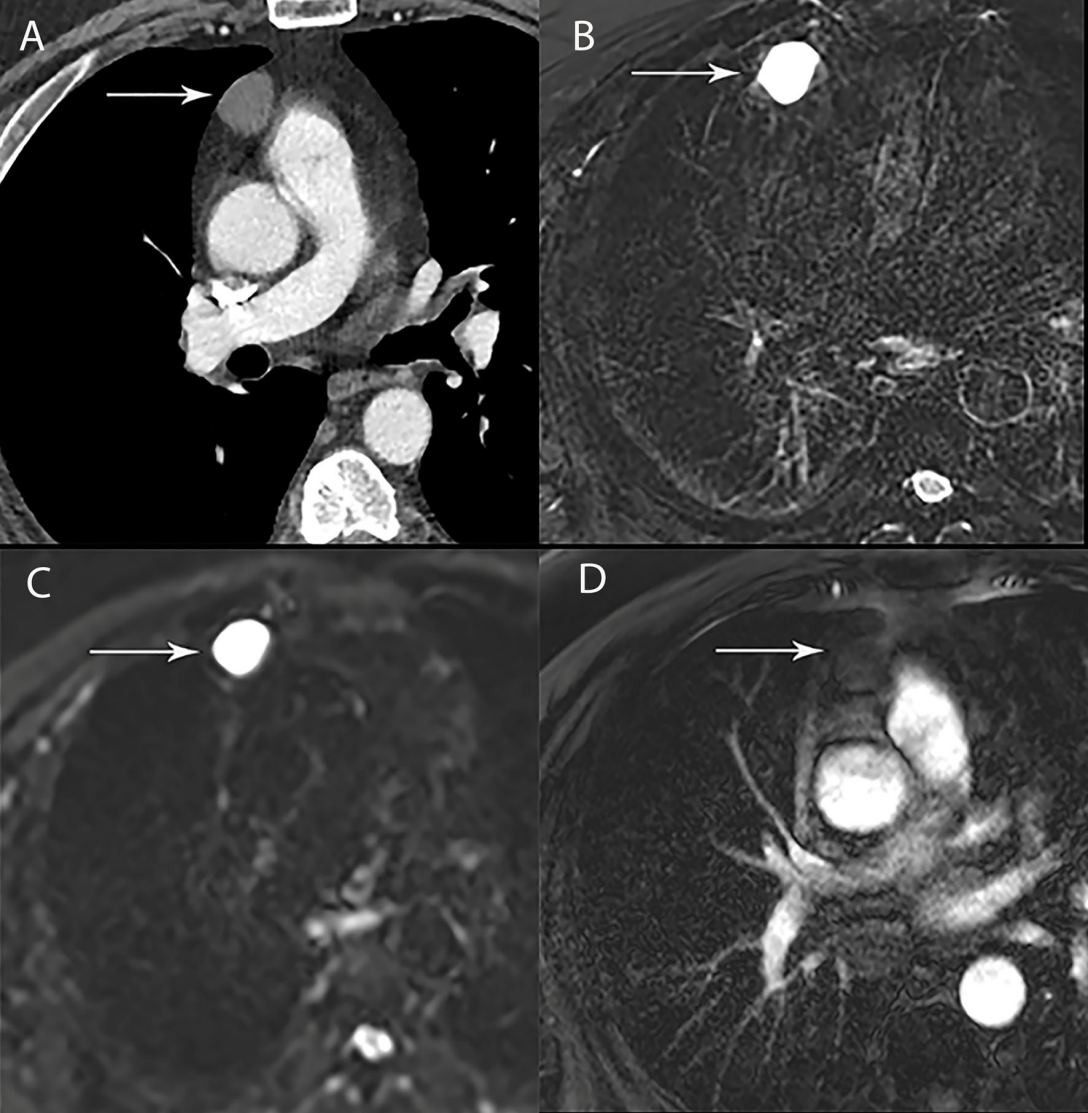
67 year old man with right thymic cyst. (A) Contrast-enhanced CT shows a right prevascular mediastinal 2.2 cm lesion (arrow) with 34 Hounsfield units, which can represent solid or cystic lesion with proteinaceous material or hemorrhage. (B–D) MRI is useful to determine that this is a simple thymic cyst (arrow) with high signal intensity on T2 weighted (B) and short tau inversion recovery (STIR) (C) and no enhancement on the post contrast images (D).
